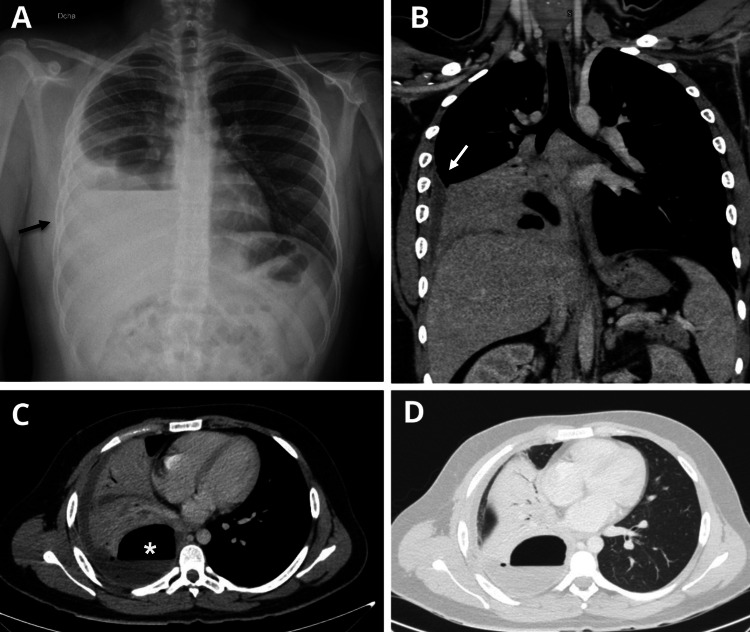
Chest radiograph showing unilateral right pleural effusion with a lenticular shape (A). In image (B), CT in the coronal view observing complex pleural effusion with pleural enhancement, laminar atelectasis (C), and empyema predominantly in the right middle lobe, occupying approximately 30% of the hemithorax (C and D), along with evidence of lower lobe pneumonia. CT: Computed tomography The black arrow shows pleural effusion on the right hemithorax in the chest radiograph. The white arrow indicates pleural enhancement. The white asterisk shows the air-fluid level on the right side of the chest.
